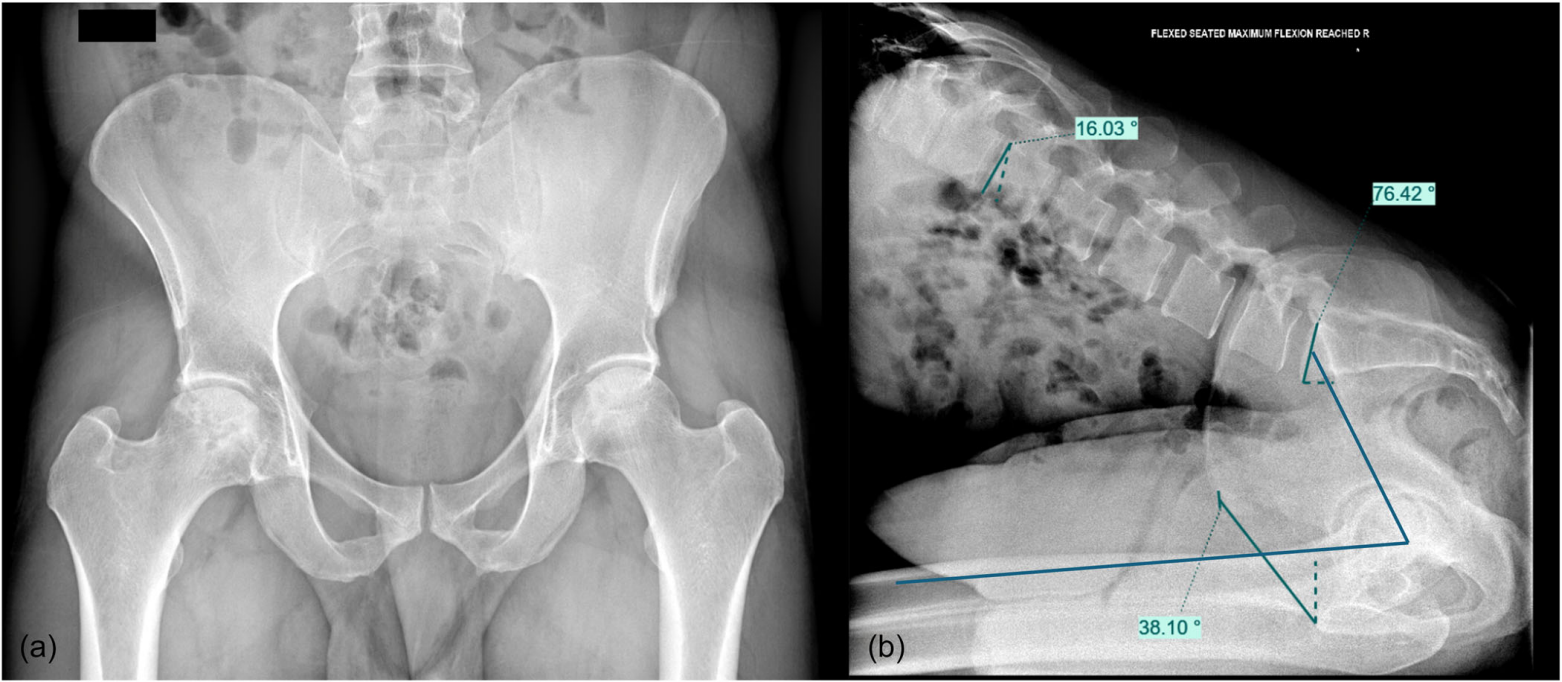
A 36‐year‐old woman with right femoral head osteonecrosis prior to THA. (a) Anteroposterior radiograph of the pelvis. (b) Lateral radiograph in the flexed‐seated position. Pelvic parameter analysis revealed no spinopelvic risk factors (spinopelvic tilt: 12°, lumbar flexion: 52°, PI: 52°, PI‐LL: −16°), but adverse spinopelvic mobility (26°) was associated with high hip mobility (∆PFA = 115°). LL, lumbar lordosis; PFA, pelvic femoral angle; PI, pelvic incidence; THA, total hip arthroplasty.
